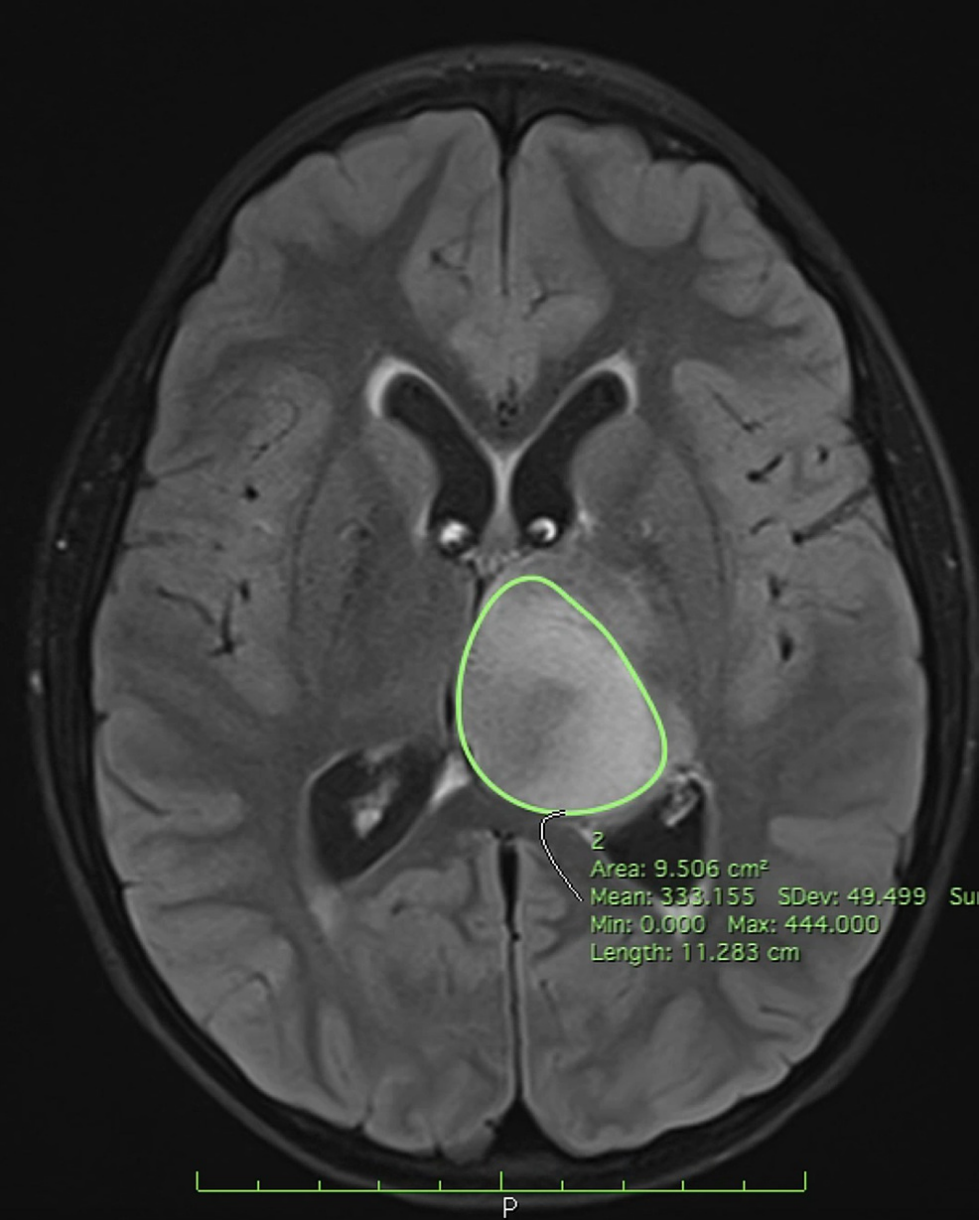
Preoperative T2 FLAIR MRI - Horos ROI segmentation Preoperative T2 FLAIR MRI demonstrating Horos region of interest (ROI) segmentation function with 3.6 cm x 3.4 cm x 4.8 cm mass of the left thalamus circumferentially outlined.
